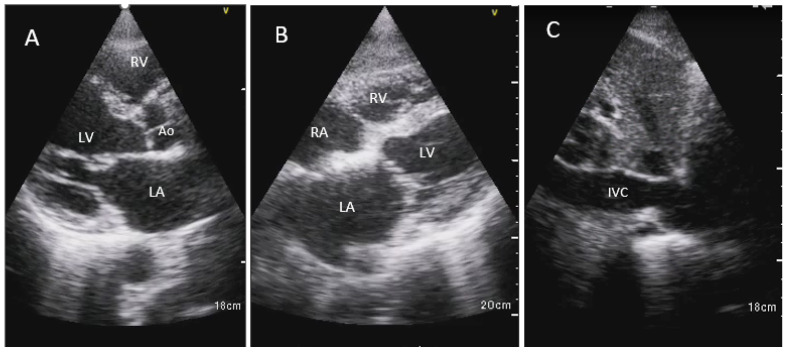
HHE images are shown in the parasternal long-axis view (A), subcostal 4 chamber view in the orthogonal plane (B), and inferior vena cava in the sagittal plane (C). Ao, aortic root; IVC, inferior vena cava; LA, left atrium; LV, left ventricle; RA, right atrium; RV, right ventricle.
